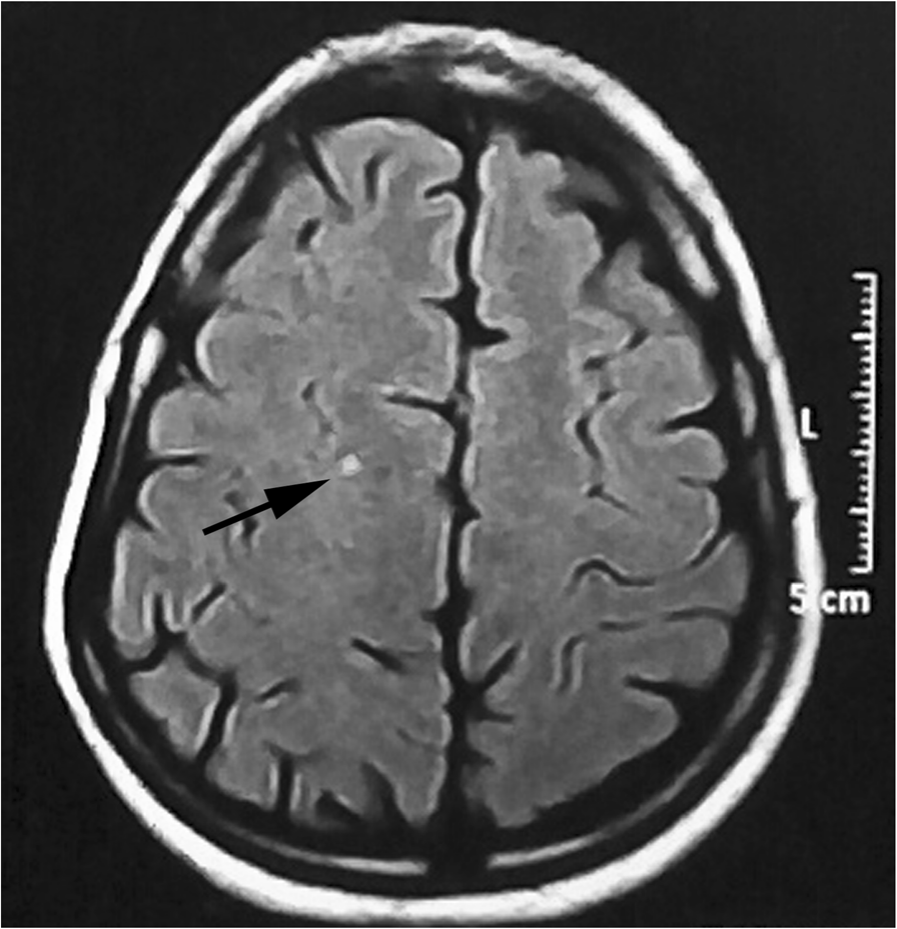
50-year-old female patient, not known to have any chronic illness, presented with migraine with aura for 10-year duration of grade III severity. Axial FLAIR MRI image shows small single bright focus at the right centrum semiovale (arrow)
